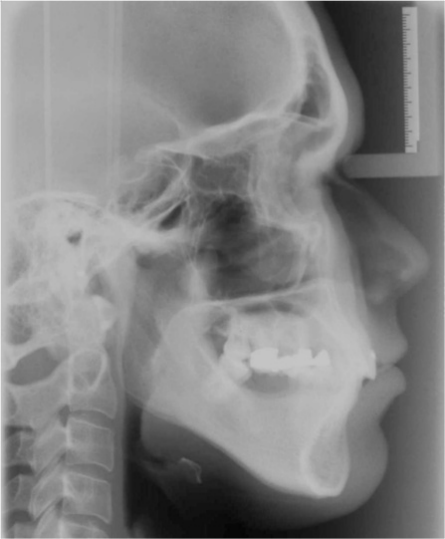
The panoramic radiography and cephalometric analysis revealed a partially edentulous mandible
